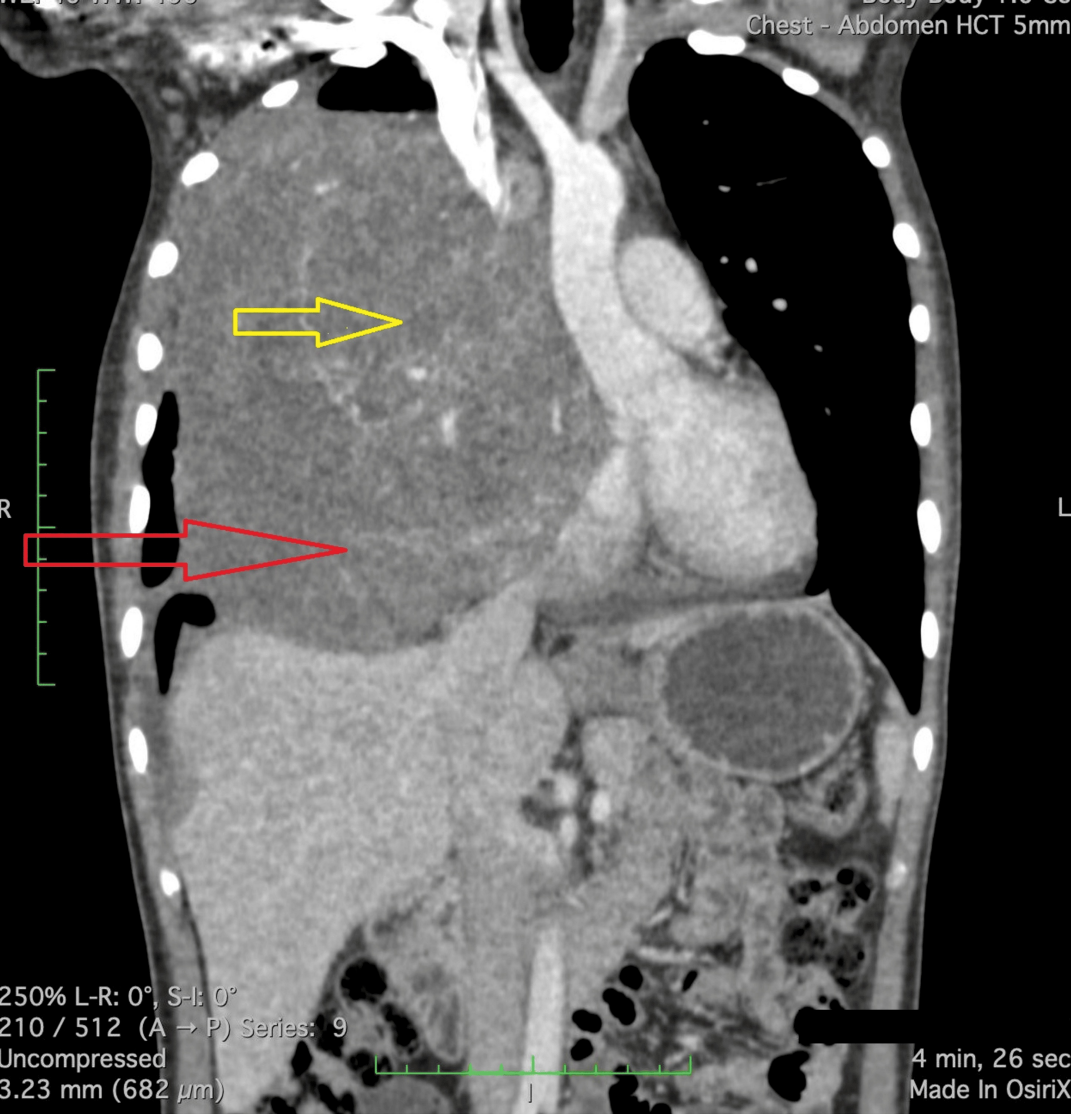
CECT Chest showing anterior mediastinal mass. Coronal CT image of the chest demonstrating a heterogeneously enhancing anterior mediastinal mass (red arrow) with a central, non-enhancing cystic or necrotic area (yellow arrow). The mass displaces adjacent structures without clear evidence of invasion. These features are consistent with a germ cell tumour, likely a yolk sac variant. CECT: contrast-enhanced computed tomography
